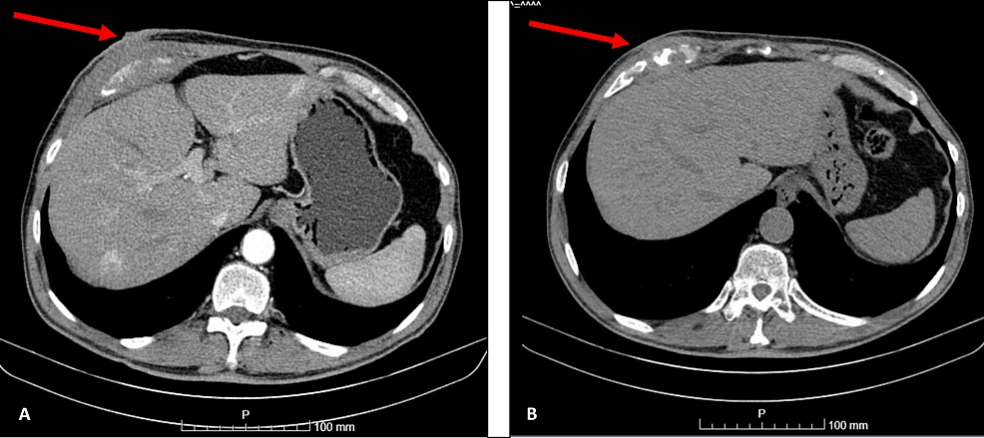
CT scan A: With intravenous contrast; B: without intravenous contrast. Red arrows show the chest wall mass.
